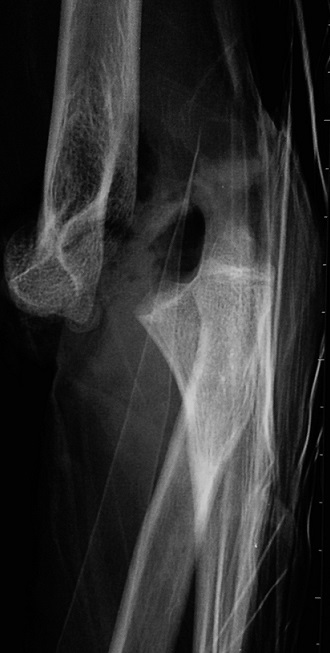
The lateral radiograph of the elbow dislocation
